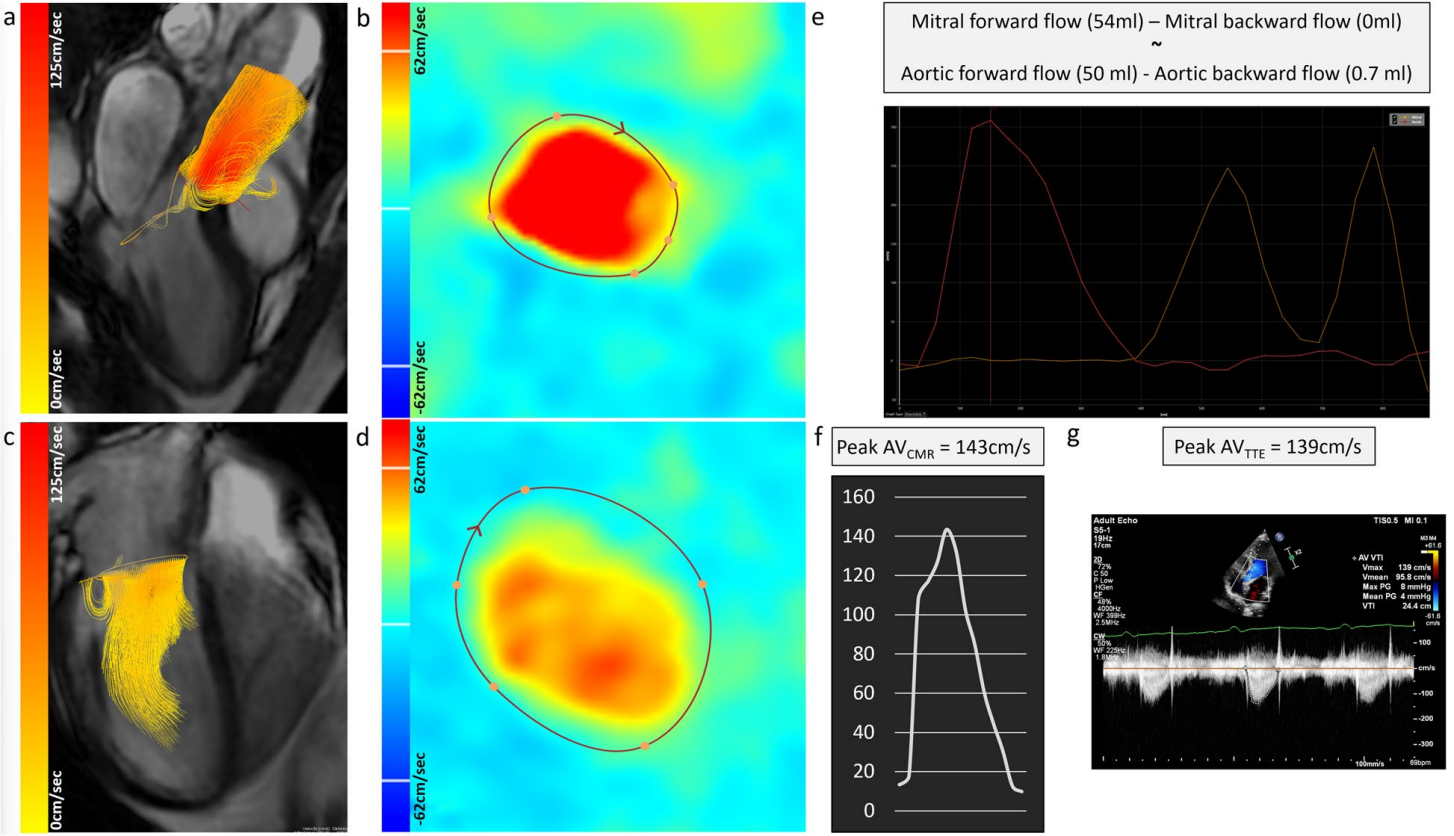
A case example from the study. Flow streamlines of aortic forward flow (a). Quantification of aortic forward flow using the reformatted phase-contrast plane (b). Mitral inflow quantification using retrospective valve tracking procedures (c, d). Demonstration of total flow and conservation of mass principle, *i.e.*, mitral forward flow (MFF) minus mitral backward flow (MBF) is equal to aortic forward flow (AFF) minus aortic backward flow ABF (e). Peak velocity through the aortic valve using three-dimensional streamlines to automatically trace the transvalvular peak (f). Echocardiography continuous Doppler method for peak velocity assessment through the aortic valve (g)
